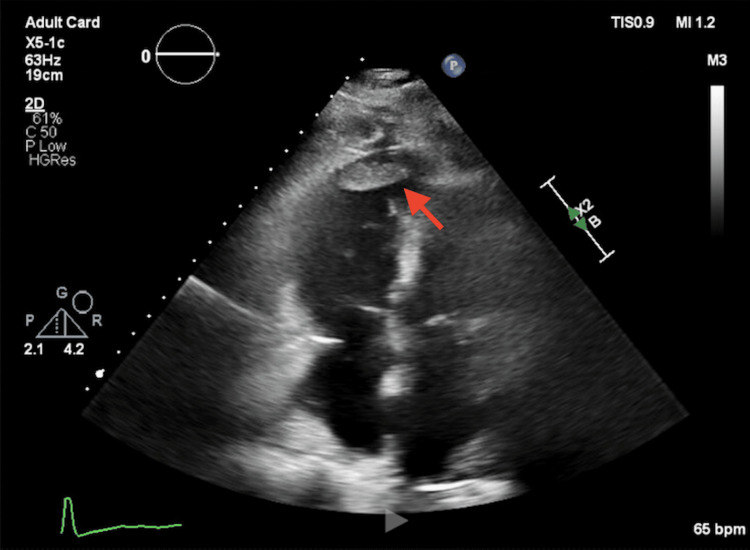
Transthoracic echocardiography showing right ventricular thrombus Transthoracic echocardiography (apical four-chamber view) demonstrates a 4.2×1.1 cm thrombus in the right ventricle (red arrow) with severely reduced right ventricular systolic function.
